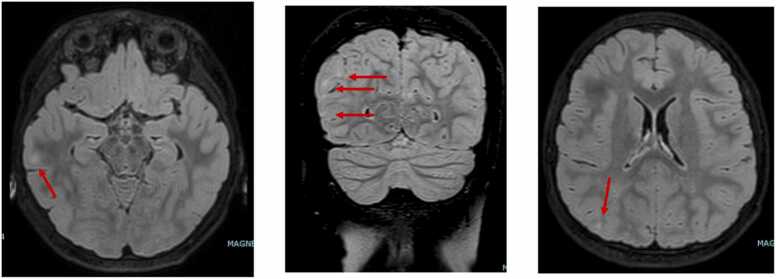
The brain MRI shows cortical and subcortical hypersignals in the right central, right temporal, and right occipital regions on the FLAIR sequence (arrows).
